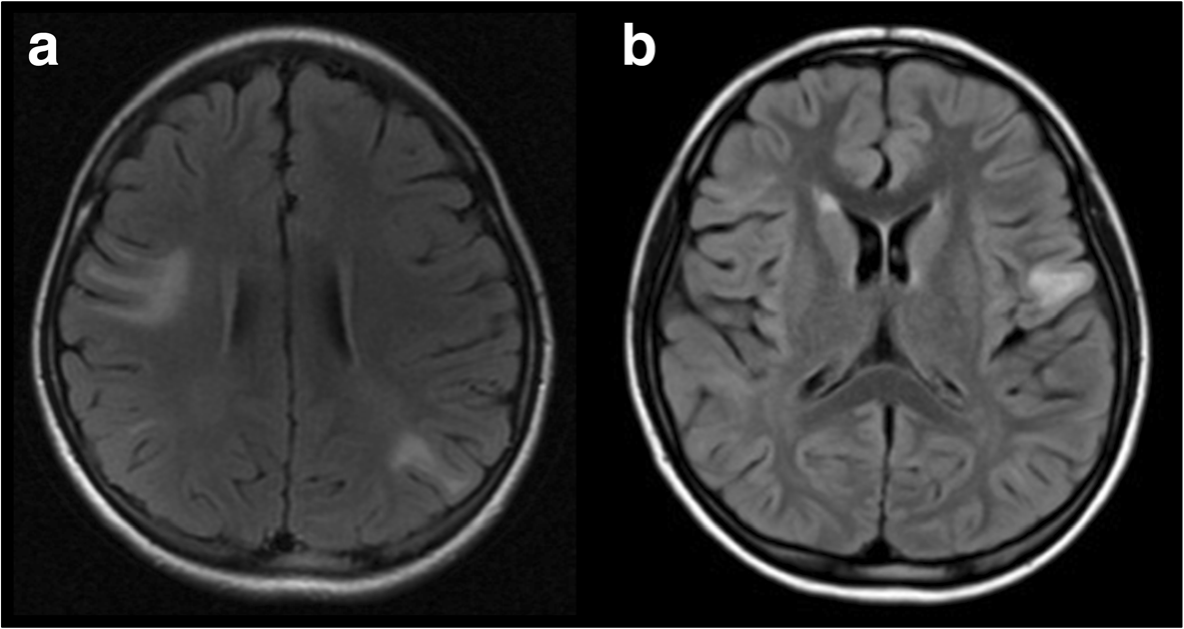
Cortex and subcortical lesions on Brain MRI. a Brain MRI on January 23, 2013: lesions in bilateral frontal lobe, left parietal lobe and right temporal lobe were seen, cortex and subcortical can be found in right frontal lobe and left parietal lobe; b Brain MRI on May 27, 2014: new lesions were observed in left frontal lobe
